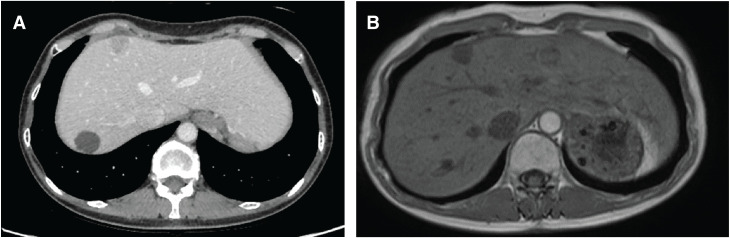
(A) CT image showing a new metastatic liver tumor in segment S4 at 10 months post-initial surgery. (B) Gd-EOB-DTPA-enhanced MRI (late phase) demonstrating the tumor’s hypervascular nature. Gd-EOB-DTPA, gadolinium ethoxybenzyl diethylenetriamine pentaacetic acid
